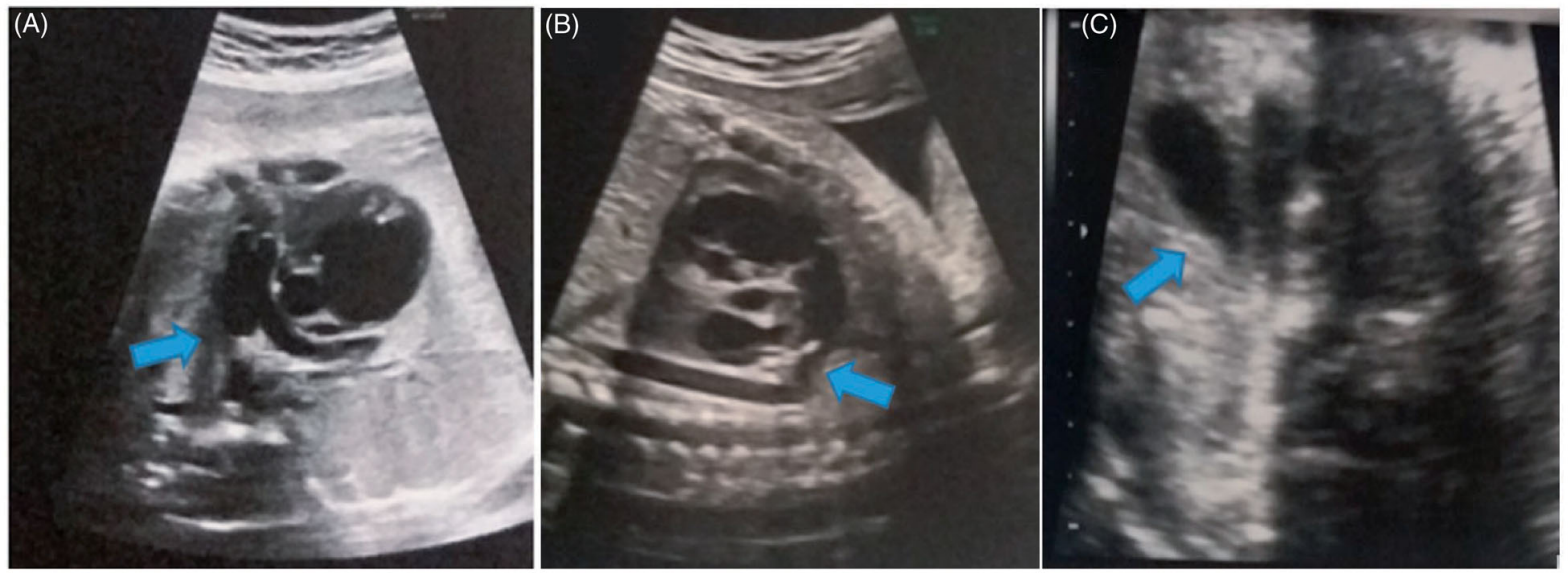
Two-dimensional echocardiography, showing ductus arteriosus constriction (arrow). (A) Right outflow tract. (B) Ductal arch view. (C) three-vessel view.
